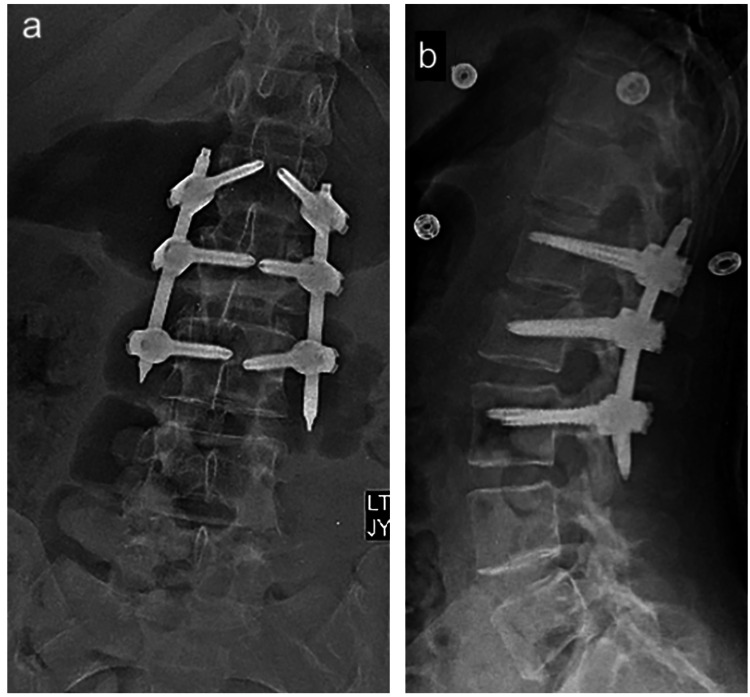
Postoperative (a) anteroposterior and (b) lateral radiographs of the lumbar spine with short-segment fixation without fusion from L1 to L3.
